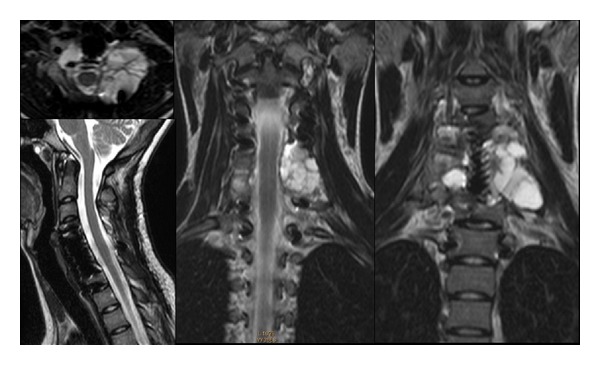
Nine-month MRI scans, showing an extensive bilateral recurrence more represented on the left side, with a characteristic “soap-bubble” appearance.
